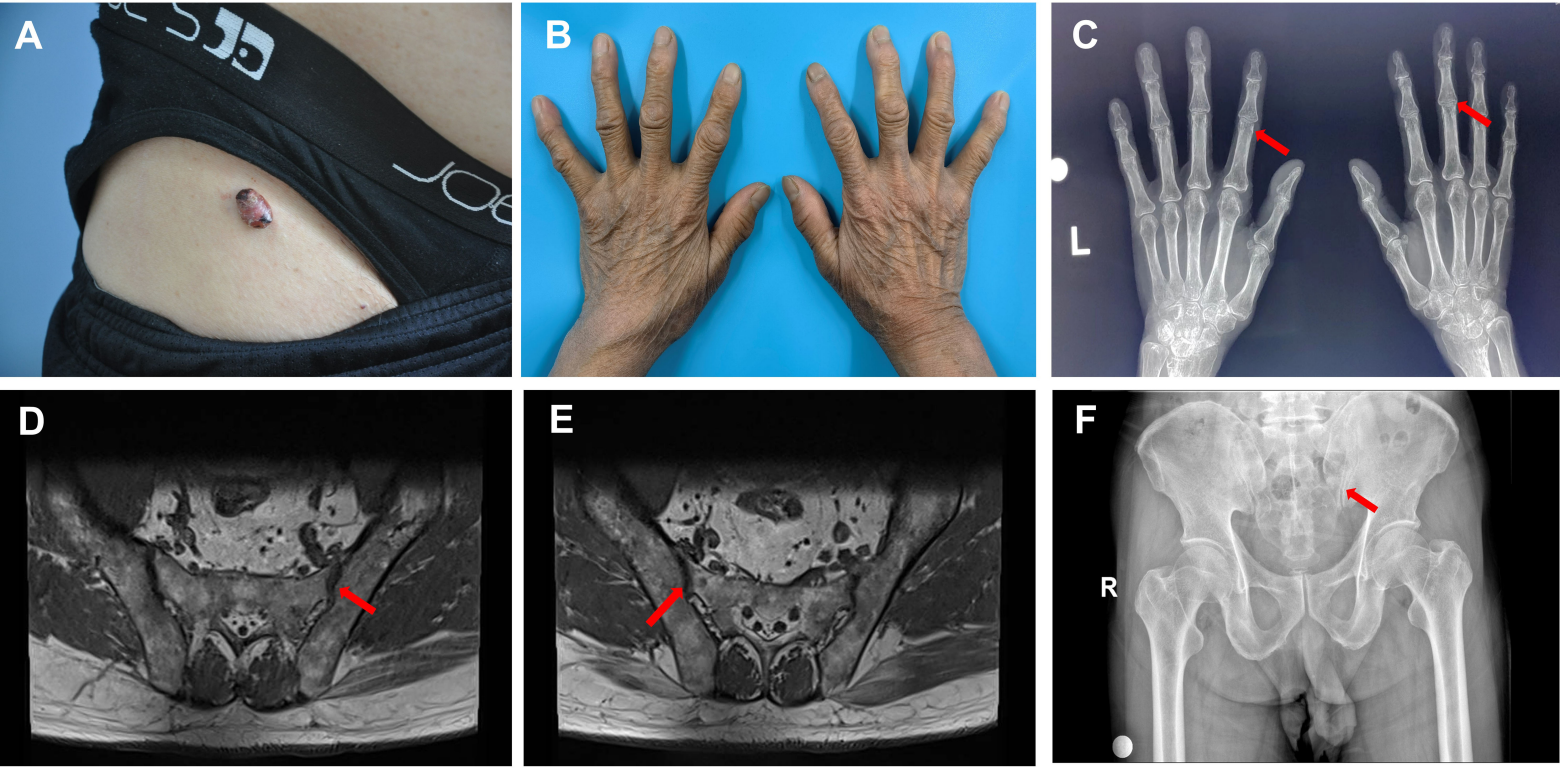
Skin changes and imaging in the patient with multicentric reticulohistiocytosis. (A) Skin lesions in the right inguinal region. (B) Mild swelling of the interphalangeal joints of both fingers of the patient, with no obvious deformation. (C) Mild bony changes at the edges of the interphalangeal joints indicated by the red arrow on the X-ray, and mild narrowing of the interphalangeal joint space. (D) MR scan of the sacroiliac joint, with the red arrow indicating lipid deposition in the hip joint. (E) MR scan of the sacroiliac joint, with the red arrow indicating lipid deposition in the hip joint. (F) Pelvic X-ray, with the red arrow indicating degenerative changes in the hip joint with decreased cystic density.
